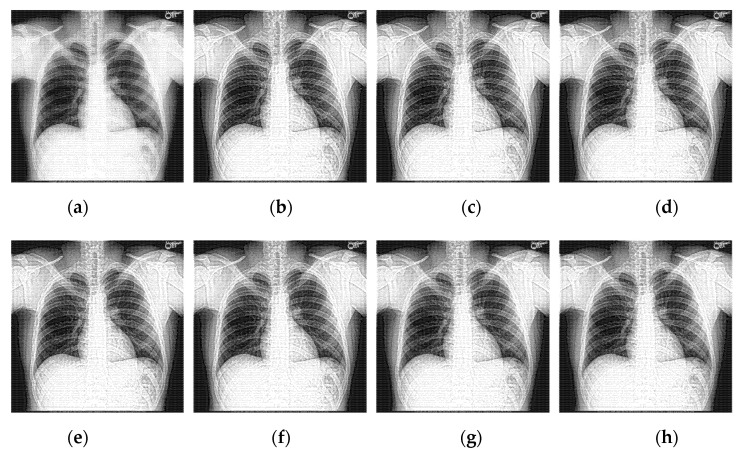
The enhanced effects of different shearing directions on X-ray image 2. In (a), the shearing parameters of each level are set to (4,4,4) and the shearing directions are (16,16,16). For (b–h), the decomposition scale was set to 4 levels, the shearing parameters of each level are set from (2,2,2,2) to (4,4,4,4), and the shearing directions are from (4,4,4,4) to (16,16,16,16). The number of shearing directions of each level was gradually increased.
